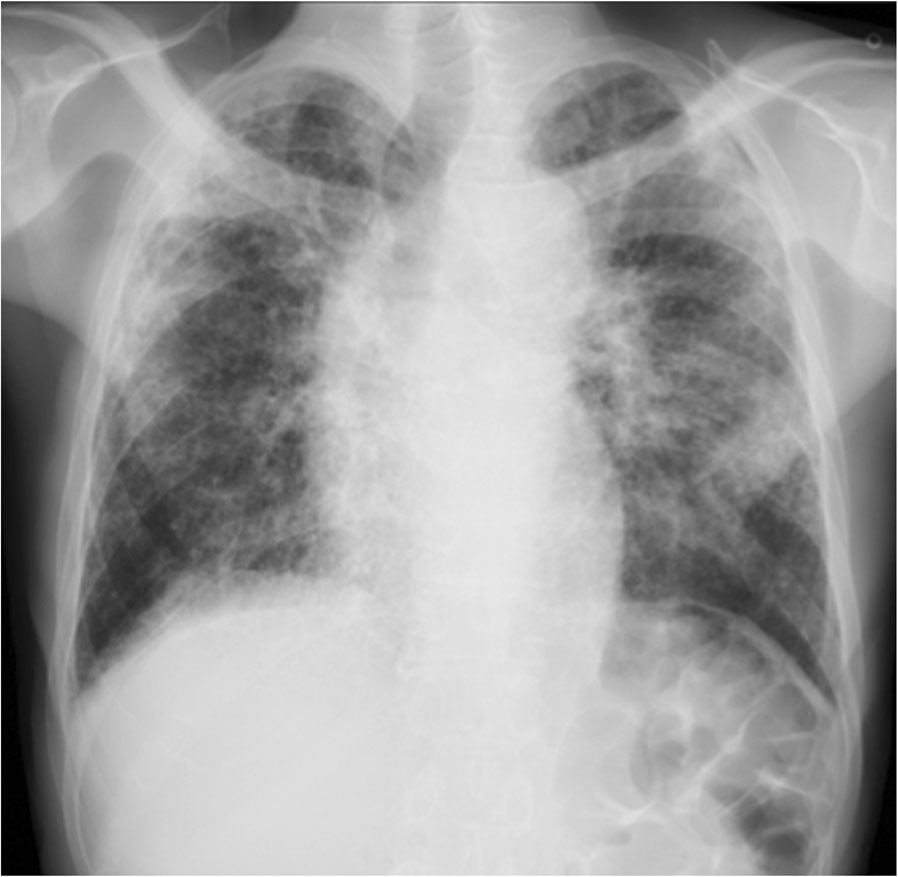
Chest X-ray showing bilateral pleural thickening in the upper and middle lung fields. The lung volume was reduced and reticulonodular shadows extended from the sub-pleura to deep inside the lungs, suggesting pulmonary fibrosis. The tracheal bifurcation was widened by traction of the upper lobes
